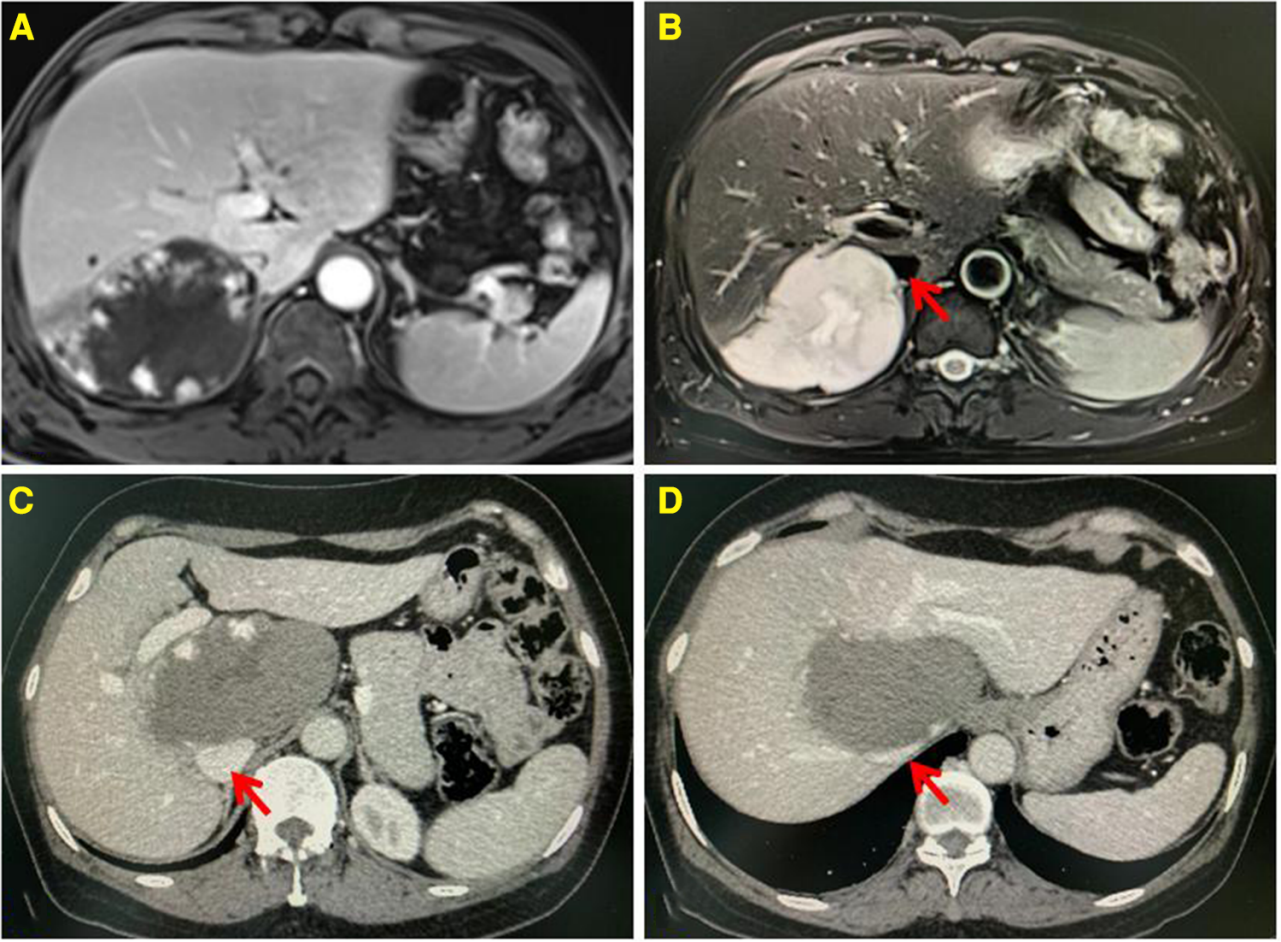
Enhanced MRI showing the lesion located in the segment VII, low signal on T1WI, and high signal on T2WI. Progressive enhancement and high strength mural nodules can be seen on the enhanced scan (A,B). Enhanced CT showing the lesion located in the segment I, having a low density. Progressive enhancement can be seen on the enhanced scan (C,D). The inferior vena cava is compressed by the lesion. The red arrow indicates the IVC.
